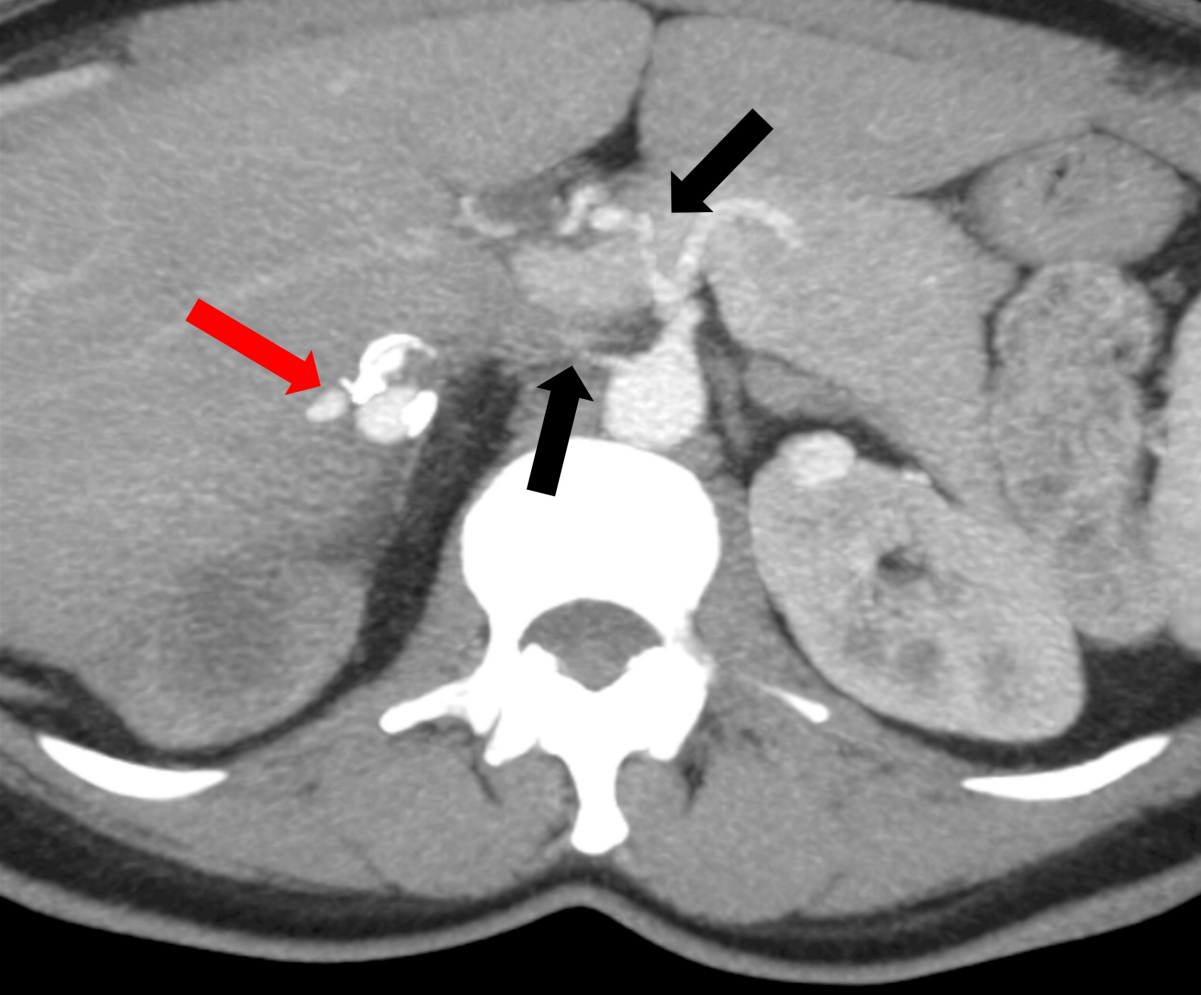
Contrast-enhanced abdominal computed tomography Two filiform renal arteries (black arrows) to right kidney, with a partially thrombosed aneurysmal (red arrow) observed.
